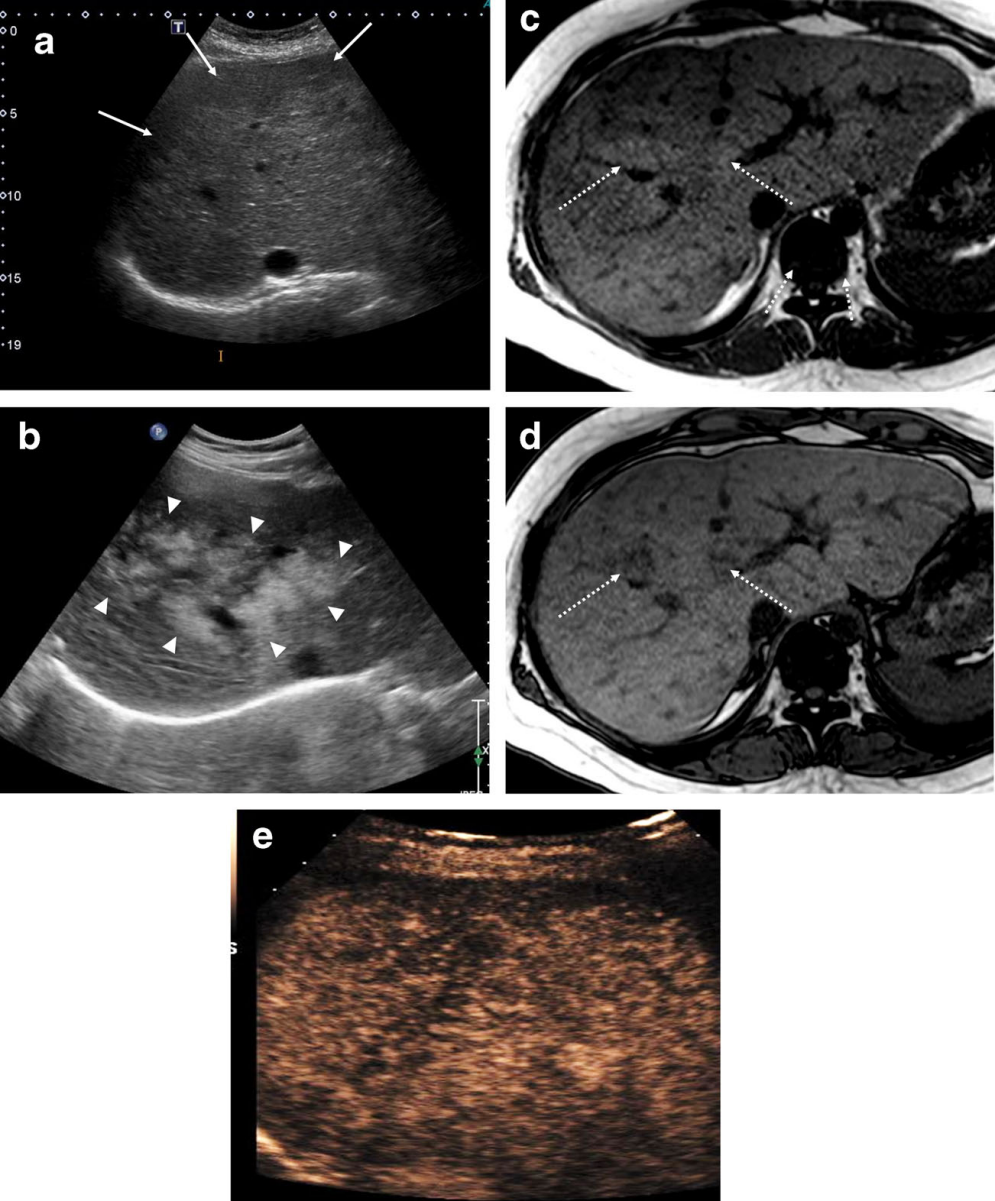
Hepatic parenchymal change in US in post-Fontan patients. a A 33-year-old woman with previous pulmonary atresia. US reveals heterogeneous parenchymal echotexture with central zone hyper-echogenicity and peripheral zone hypo-echogenicity (*arrows*). b-d A 30-year-old woman with previous hypoplastic right ventricle syndrome. b US shows irregular homogeneous hyperechoic mass-like areas (*arrowheads*) surrounding portal vein branches with geographic margin. c, d In-phase and opposed-phase axial T1-weighted MRI demonstrates increased signal intensity (SI) on in-phase image (c) and dropped SI on opposed-phase image (d), suggesting fat deposit (*dotted arrows*). These areas correspond to the hyperechoic mass-like areas on US. e A 48-year-old woman with previous double outlet right ventricle. Contrast-enhanced ultrasound image obtained at 3 min shows marked coarse and heterogeneously weak delayed parenchymal enhancement
